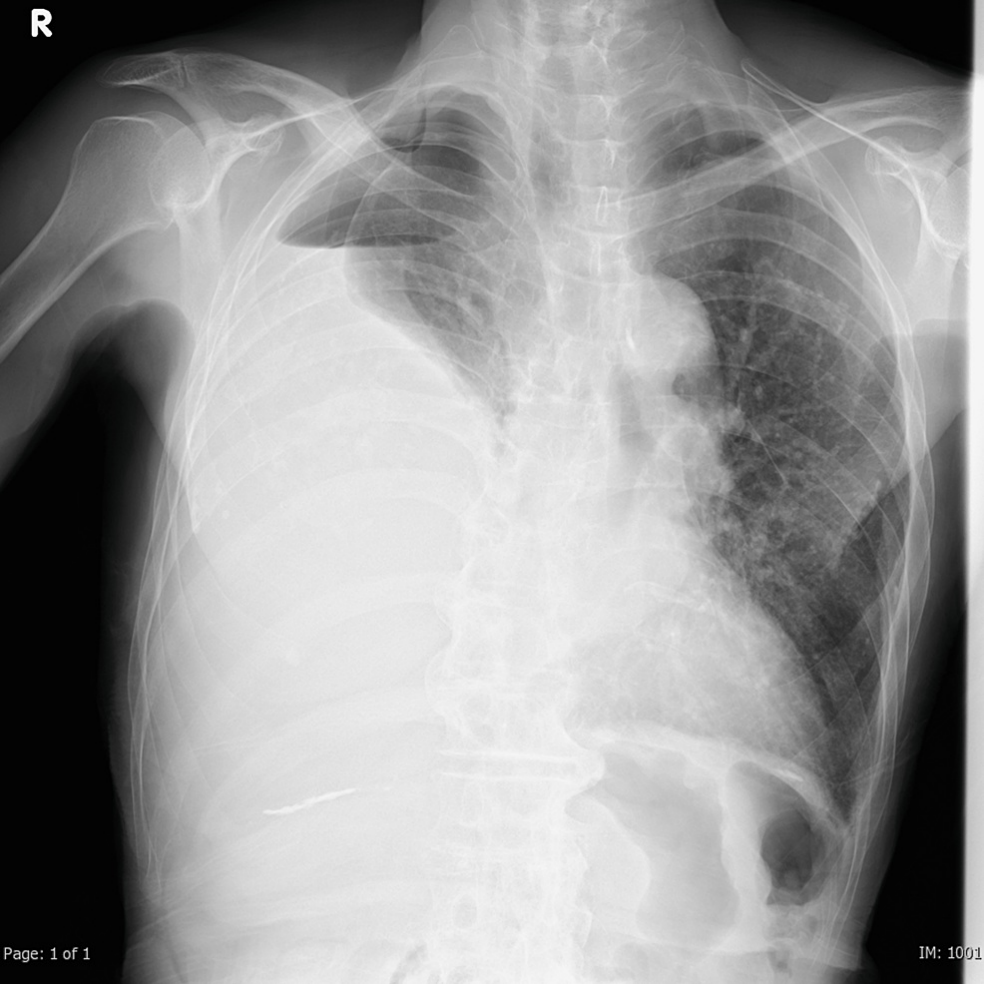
Chest radiography revealed reduced translucency and a pneumothorax in the right lung field.
